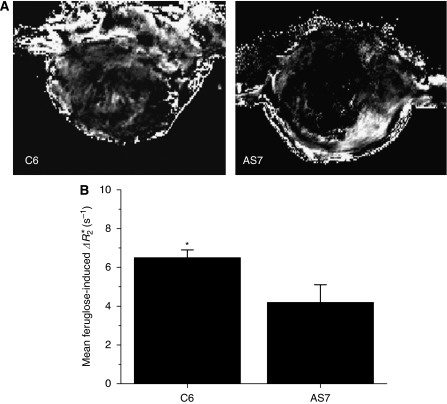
Effect of antisense iNOS on tumour blood volume assessed by MRI. (A) Calculated Δ*R*_2_^*^ maps of C6 and AS7 tumours. Note the dark core within the core of the map of the AS7 tumour, indicating an absence of feruglose uptake within these regions. (B) Summary of feruglose-induced Δ*R*_2_^*^ of parental C6 (*n*=6) and AS7 (*n*=7) tumours. Results are mean±1 s.e.m. ^*^*P*<0.05, Student's *t*-test.
